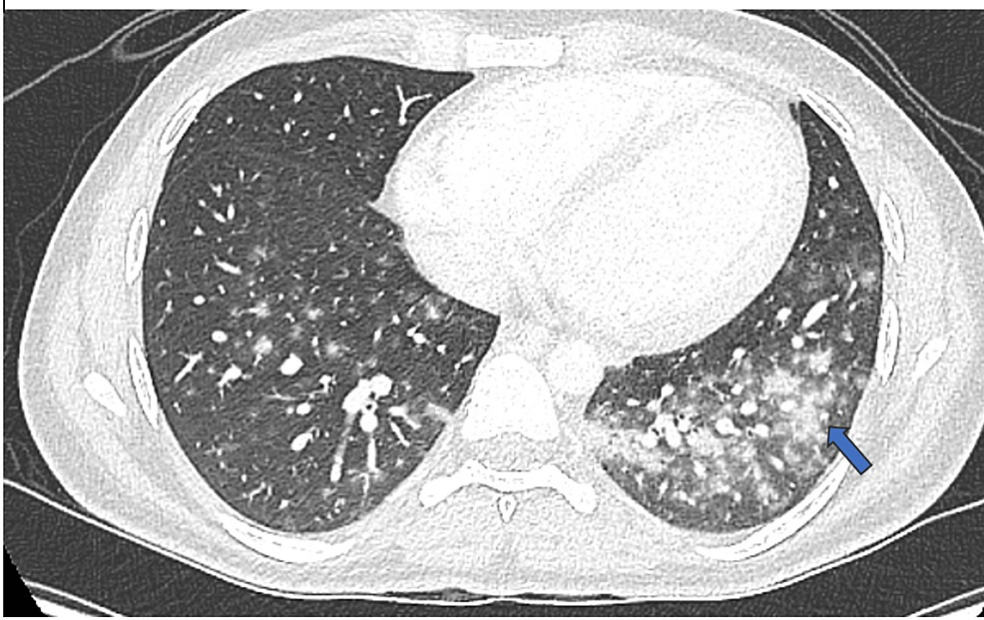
High-resolution computed tomography (HRCT) of the lungs showing large clusters of centrilobular nodules noted within both lower lobes.
